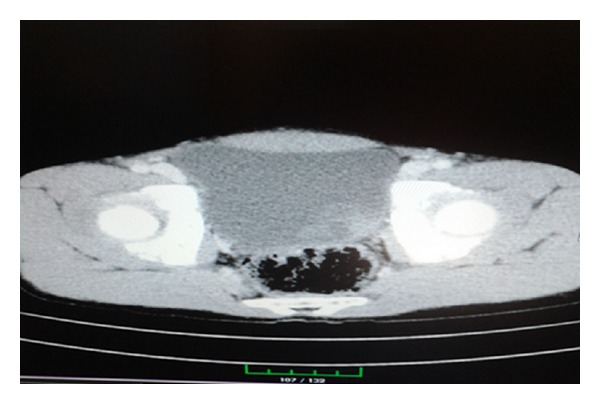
On contrasted CT, a suspicious lesion with thin septa was observed at posterolateral wall of bladder (3.5 × 3 cm in size).
